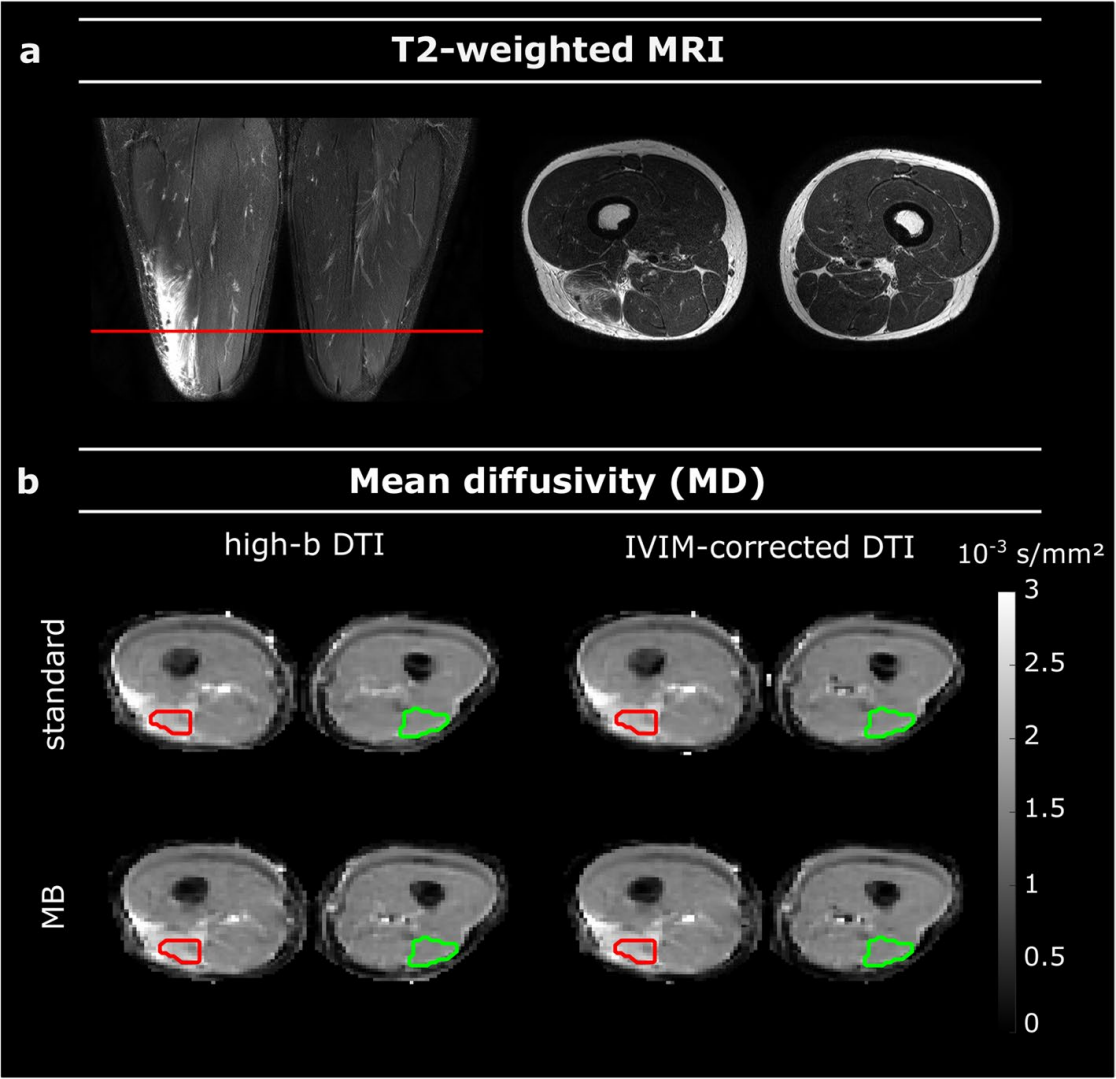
Representative images of an athlete with grade 2 injury in the right biceps femoris long head muscle. a T2-weighted images used for injury grading. The red line indicates the slice identified by a radiologist as containing the primary location of the injury. The axial image of this slice is shown. b Parameter maps of the same axial slice of the MD at baseline with both fitting methods (high-*b* DTI and IVIM-corrected DTI) as well as for the standard and MB-accelerated scans. The segmentations of the injured and healthy muscles are overlaid in red and green, respectively. *MD*, Mean diffusivity; *MB*, Multiband
